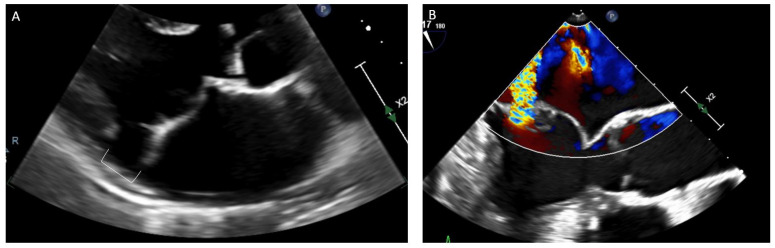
Echocardiographic findings of arrhythmic mitral valve prolapse and mitral annular disjunction. (A) Transthoracic echocardiography view of bileaflet prolapse seen with mitral annular disjunction (bracket) in the parasternal long axis view. (B) Transesophageal echocardiography view of mitral valve prolapse with color Doppler showing mitral regurgitation in a mid-esophageal long axis view.
